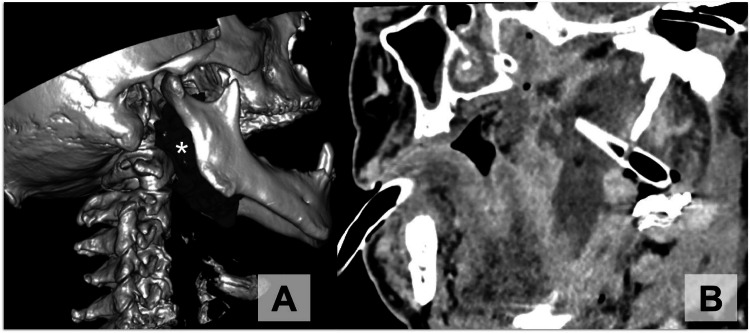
Temporal bone CT 3D reconstruction and drain position scans (A) The patient's temporal bone CT 3D volume-rendering reconstruction using the region-of-interest (ROI) technique. The total volume of 40 cm of the abscess (asterisk) was calculated preoperatively. (B) Oblique sagittal reformat of the patient's CT scan after surgical drainage showing the exact position of the drain in the abscess cavity.
